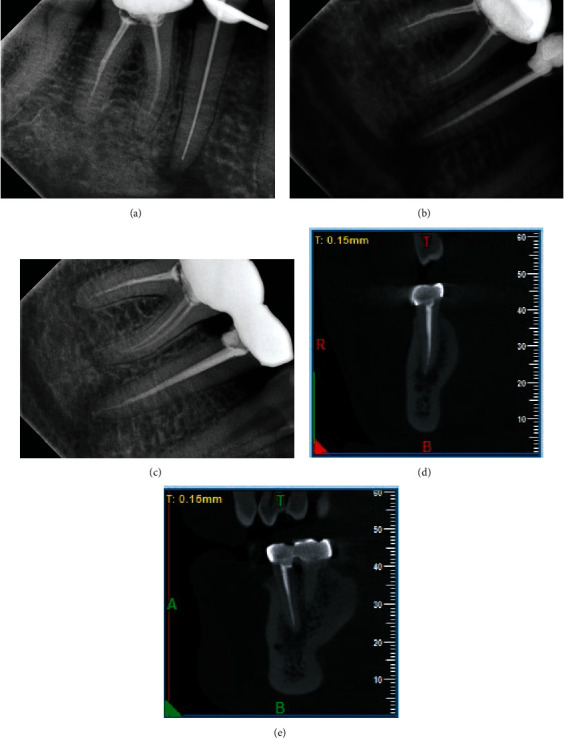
(a) Working length determination, (b) obturation, (c) 3-month follow-up RVG, and (d, e) 12-month follow-up CBCT.
